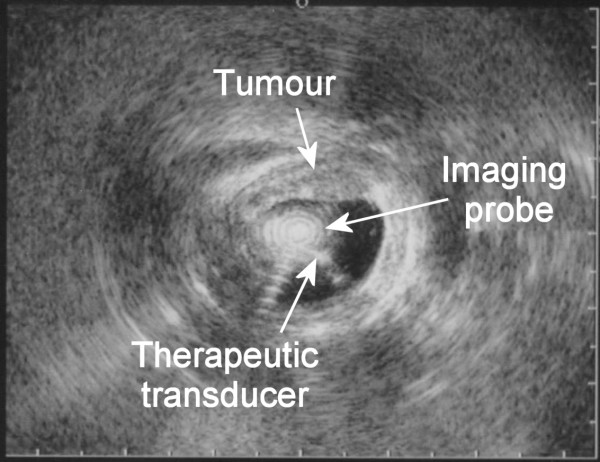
Sonogram of an esophageal tumor obtained during treatment.
